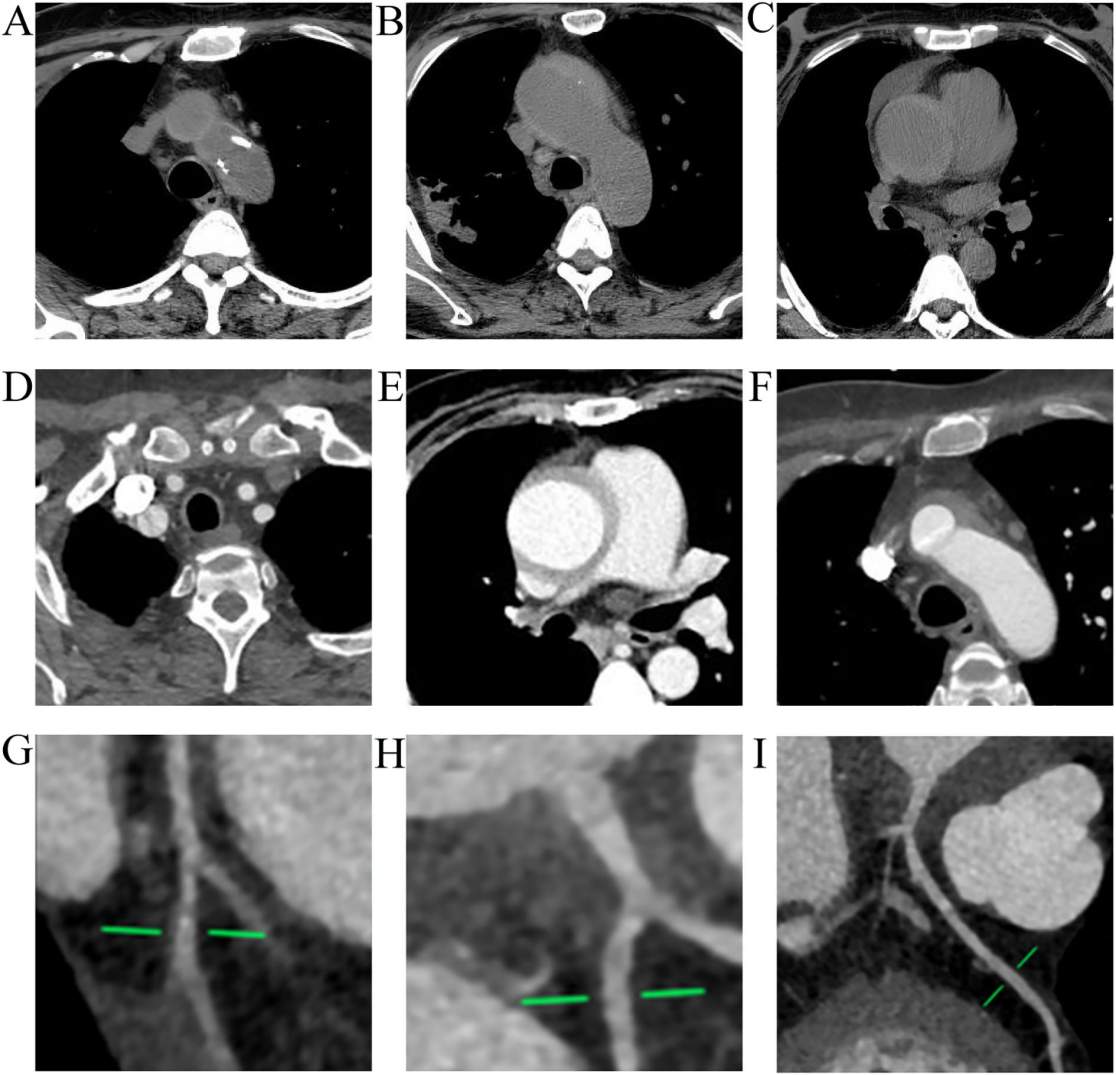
Whole-aorta CTA and coronary CTA prior to the index acute coronary event. (A–F) Whole-aorta computed tomography angiography (CTA) revealed Stanford type A aortic pathology, with mural abnormalities consistent with an intramural hematoma and/or dissection involving the ascending aorta and aortic arch, with extension to the brachiocephalic (innominate) artery origin. (D) shows the brachiocephalic artery origin; panels (A, B, F) show the aortic arch; and (C, E) show the ascending aorta. (G–I) Coronary CTA obtained during the same evaluation. (G, H) depict the left circumflex artery (LCx) and its branches, and (I) depicts the left main (LM) and left anterior descending (LAD) arteries. Although the initial CTA report did not identify focal obstructive stenosis, a retrospective review demonstrated subtle diffuse tapering of the LM (green markers), which later correlated with severe LM involvement on invasive coronary angiography during NSTEMI admission. ACS, acute coronary syndrome; CTA, computed tomography angiography; IMH, intramural hematoma; LAD, left anterior descending artery; LCx, left circumflex artery; LM, left main coronary artery; NSTEMI, non–ST-segment elevation myocardial infarction; RCA, right coronary artery.
